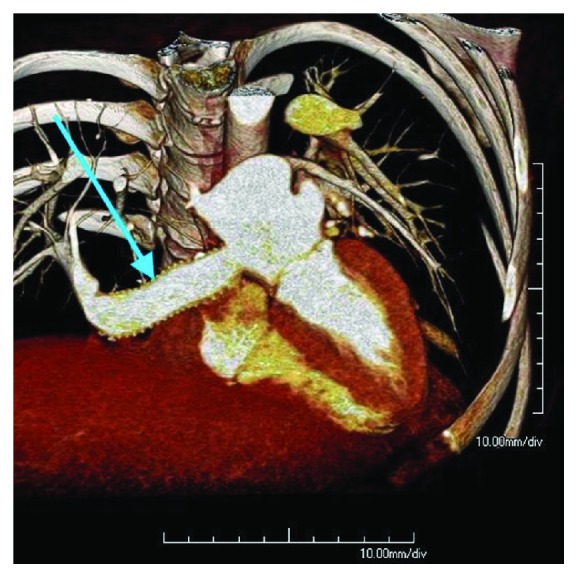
3D volume rendering, cutaway view, of postoperative CTA. Arrow pointing to the artificial baffle connecting the scimitar vein with the left atrium via the posterior aspect of the right atrium.
